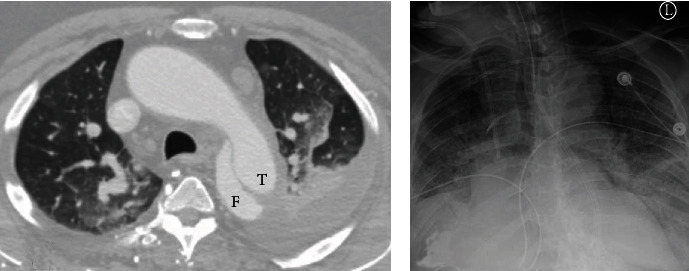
(a) CTA of chest (February 16, 2020) with Type B aortic dissection (T: true lumen; F: false lumen). (b) Chest X-ray (February 28, 2020) with bilateral pulmonary opacities and pleural effusions.
